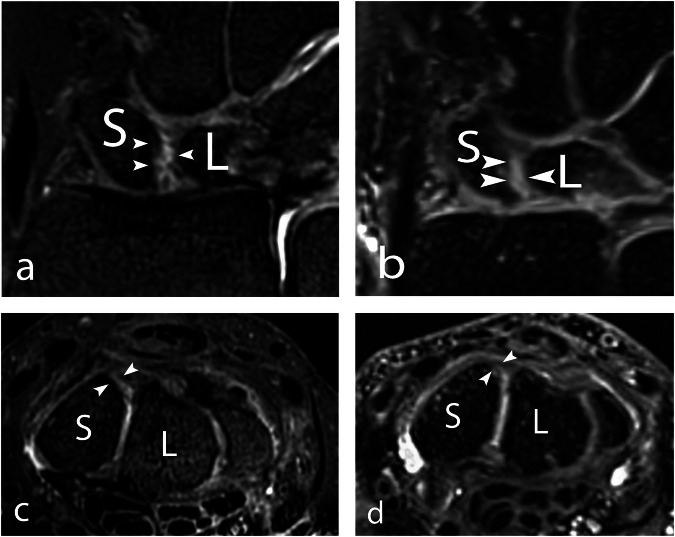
A 52-year-old male with left-sided wrist pain after a work-related injury. A subtle dorsal SLL injury (arrowheads) can be discerned on coronal and axial reformations at (a, c) 7-T and (b, d) 3-T 3D PD-weighted MRI. Multiplanar reformation was necessary to visualize this injury. SLL, scapholunate ligament; T, Tesla; S, scaphoid; L, lunate
